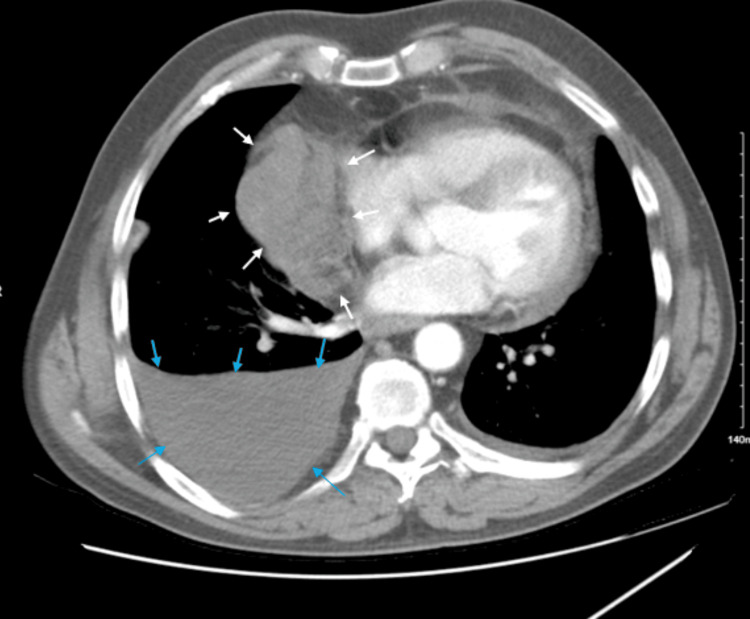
CT scan of the chest showing ‘soft tissue mass’ shown by the white arrows and the ‘pleural effusion’ noted by the blue arrows. CT: computed tomography.
